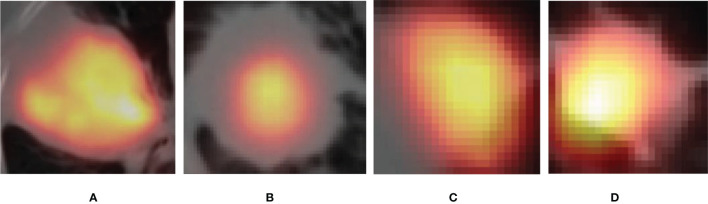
The selected four samples of (A) ADC, (B) SCC, (C) ASC, and (D) BID in PET/CT images.
